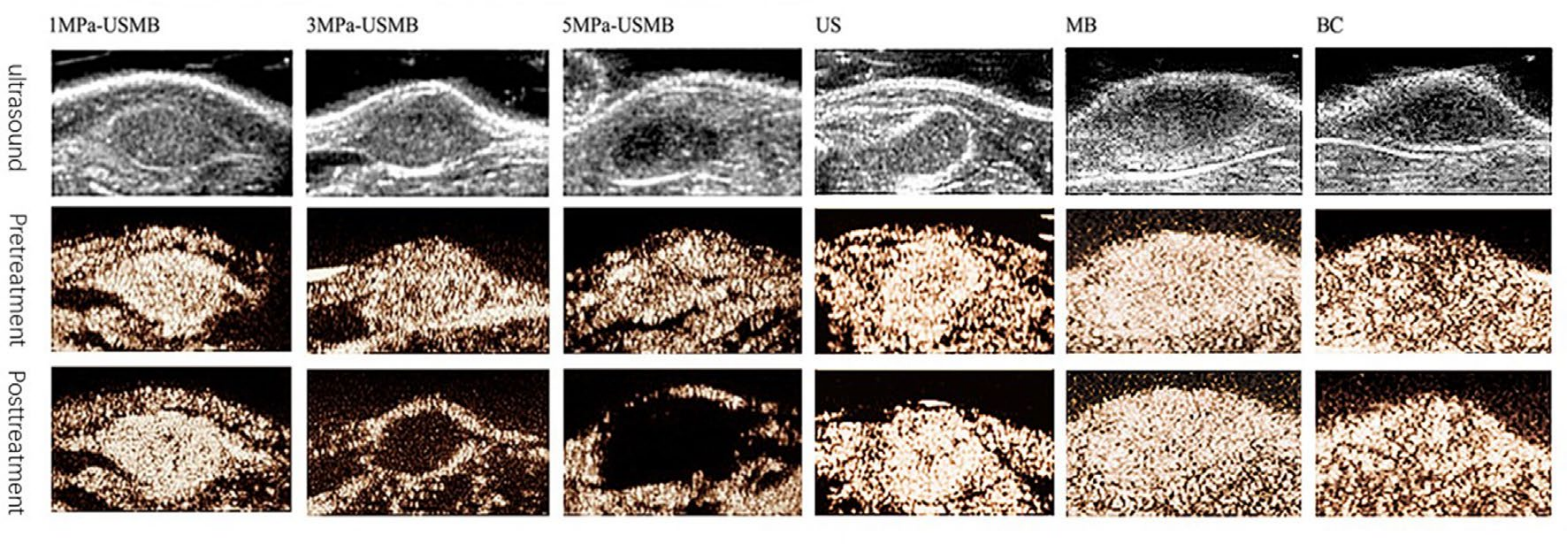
Microbubbles were filled into the tumor rapidly and evenly, and no filling defect was found in all groups before treatment. After treatment, enhancement of the tumor was marginally stronger than pretreatment in low-intensity ultrasound combined with microbubble (1MPa-USMB) group, but in medium-intensity ultrasound combined with microbubble (3MPa-USMB) group, the contrast-enhanced ultrasound (CEUS) showed a filling defect in the center of the tumor with a ring-shaped enhancement around the tumor, and the filling defect was larger in high-intensity ultrasound combined with microbubble (5MPa-USMB) group than in 3MPa-USMB group. The blood perfusion after treatment in US group, MB group, and BC group were similar, and the microbubbles were filled into the tumor quickly and completely.
